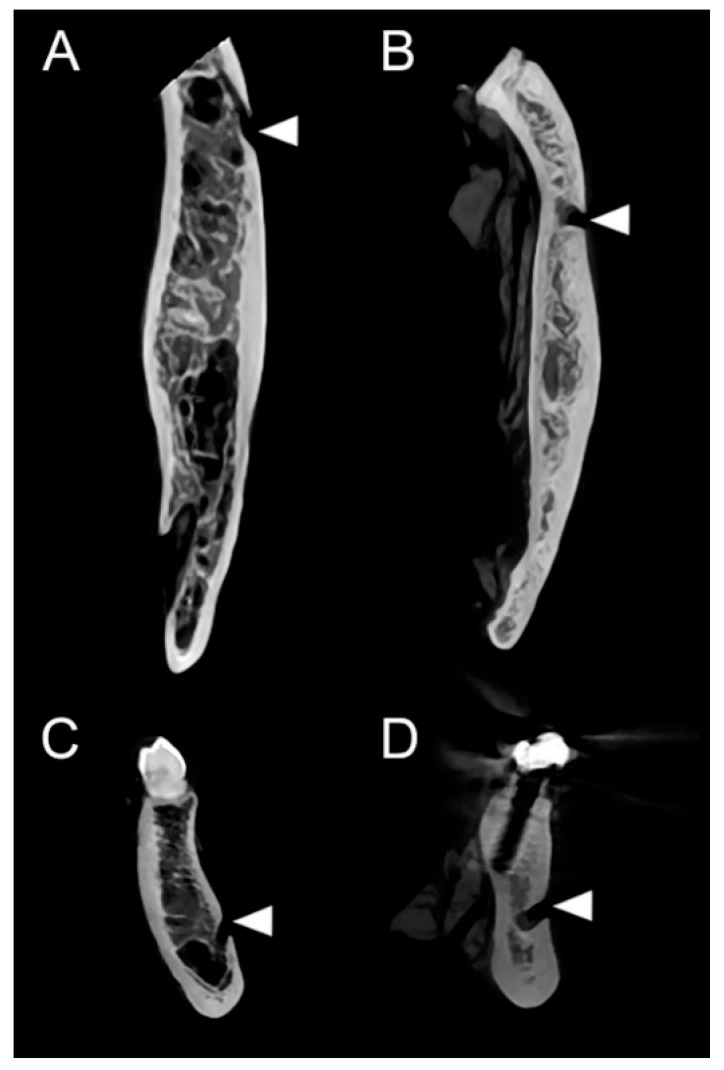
CBCT-based analysis of the opening direction of the mental canal. (A,C) Axial (A) and coronal (C) images demonstrating the posterolateral opening type, with the mental canal coursing posterolaterally toward the mental foramen (MF). (B,D) Axial (B) and coronal (D) images demonstrating the lateral opening type, in which the mental canal courses almost laterally before exiting through the MF. Arrowheads indicate the opening of the mental canal.
